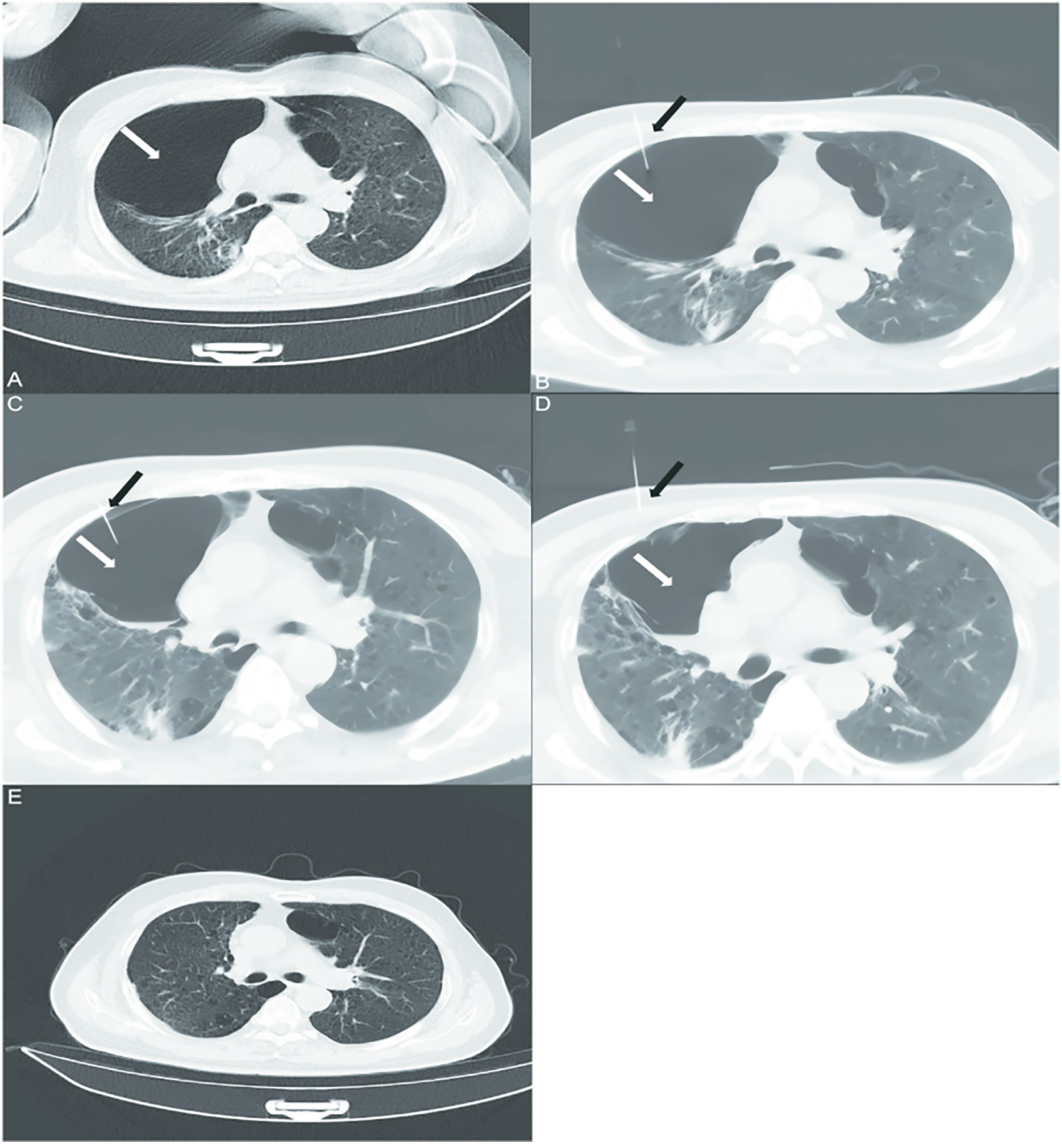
Axial computed tomography scans of the chest in patients with bilateral emphysema and alveoli before and after treatment with percutaneous bullae reduction surgery. (A) Chest CT shows emphysema and pulmonary bullae in both lungs(white arrows). (B–D) A puncture needle (black arrows) was inserted into the patient’s lung cavity on 3 consecutive days, and the air was aspirated and injected with polidocanol. (E) On follow-up chest CT at 3 months of treatment, the patient’s pulmonary alveoli showed significant improvement.
